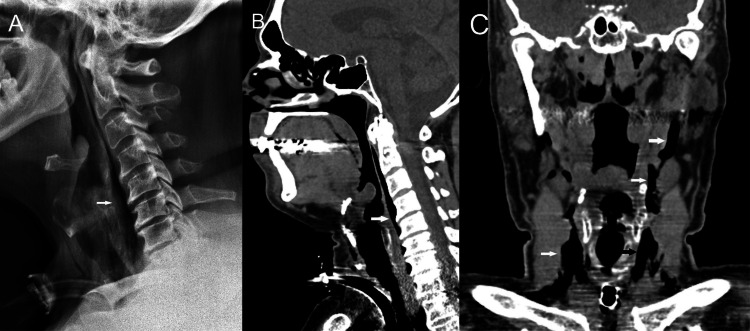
Scans of a 61-year-old man intubated due to a serious COVID-19 lung infection The lateral radiograph (A) and reformatted sagittal (B) and axial (C) CT images show craniocervical pneumatosis (arrows).
